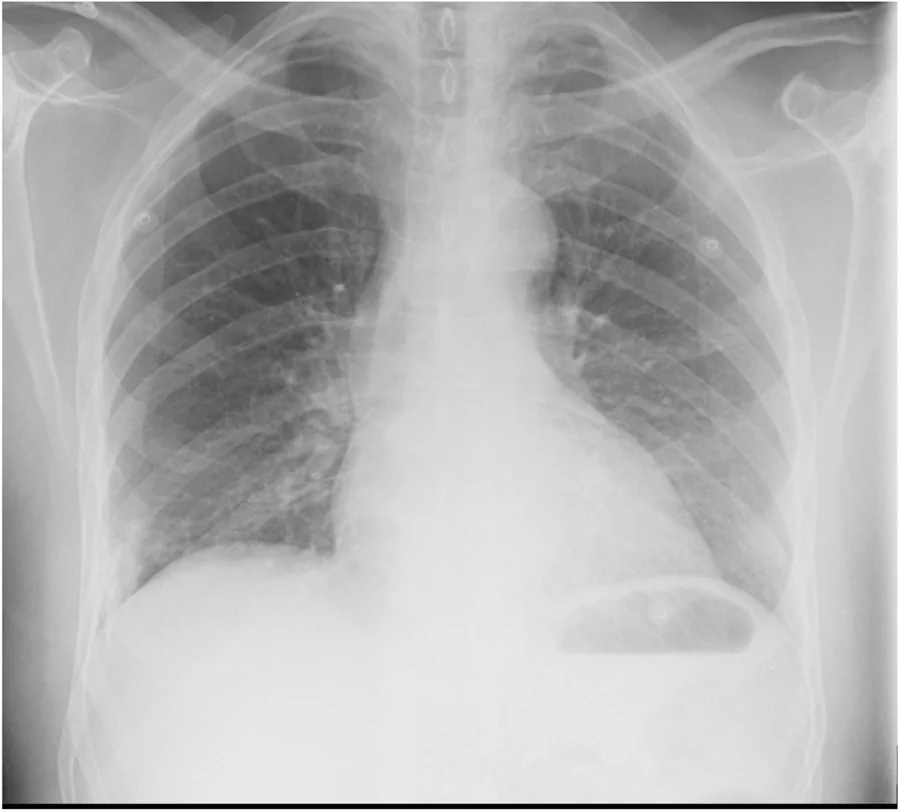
Example chest X-ray image. Cite mimic cxr V2.1.0.
